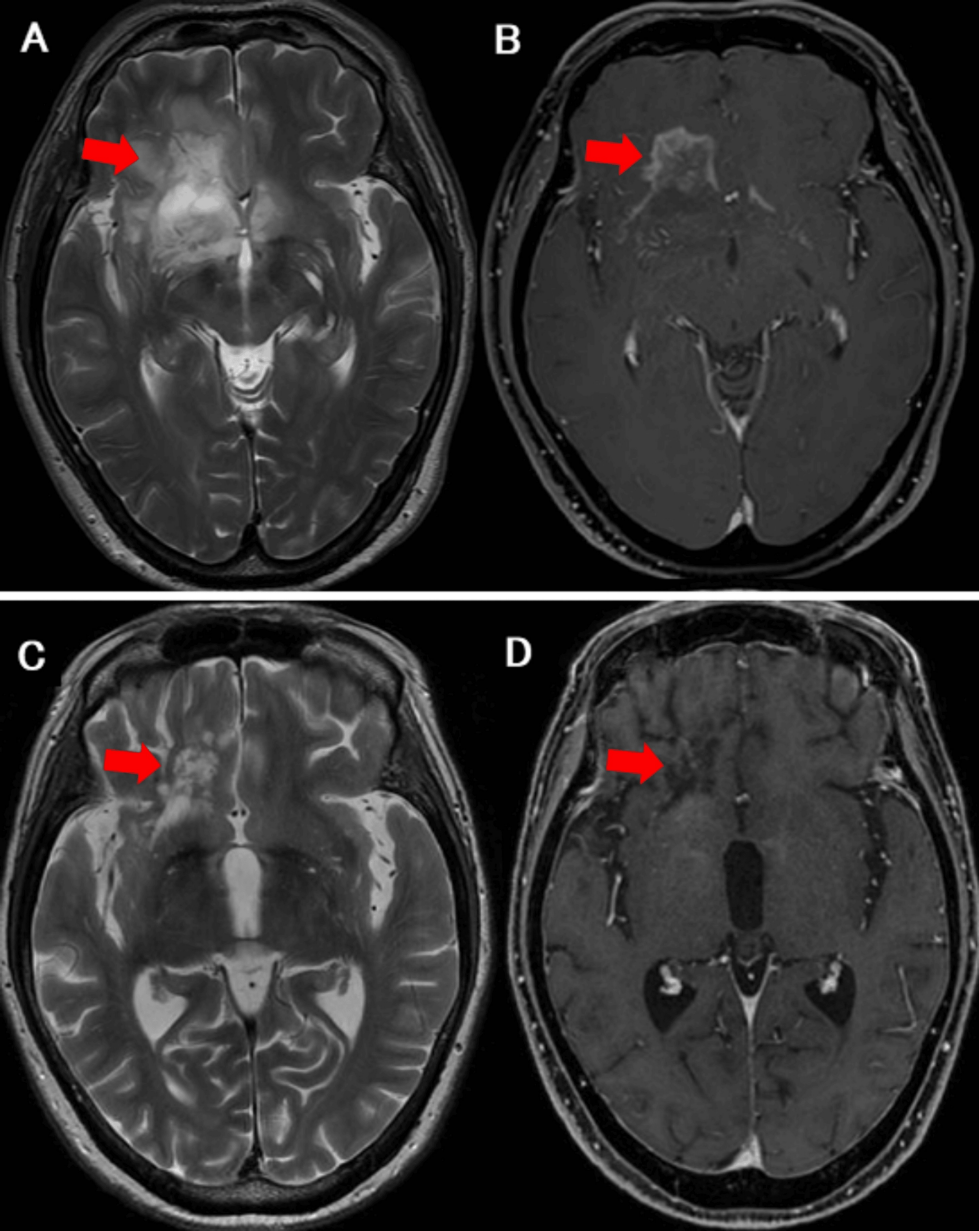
Contrast-enhanced brain magnetic resonance imaging before and after treatment A contrast-enhanced brain magnetic resonance imaging (MRI) showed a high-signal area (A) on the T2-weighted image and a corresponding enhancing lesion in the white matter of the frontal lobe (B). After 36 days of prednisolone therapy, a follow-up MRI showed marked improvement in the brain parenchymal lesion (C and D).
